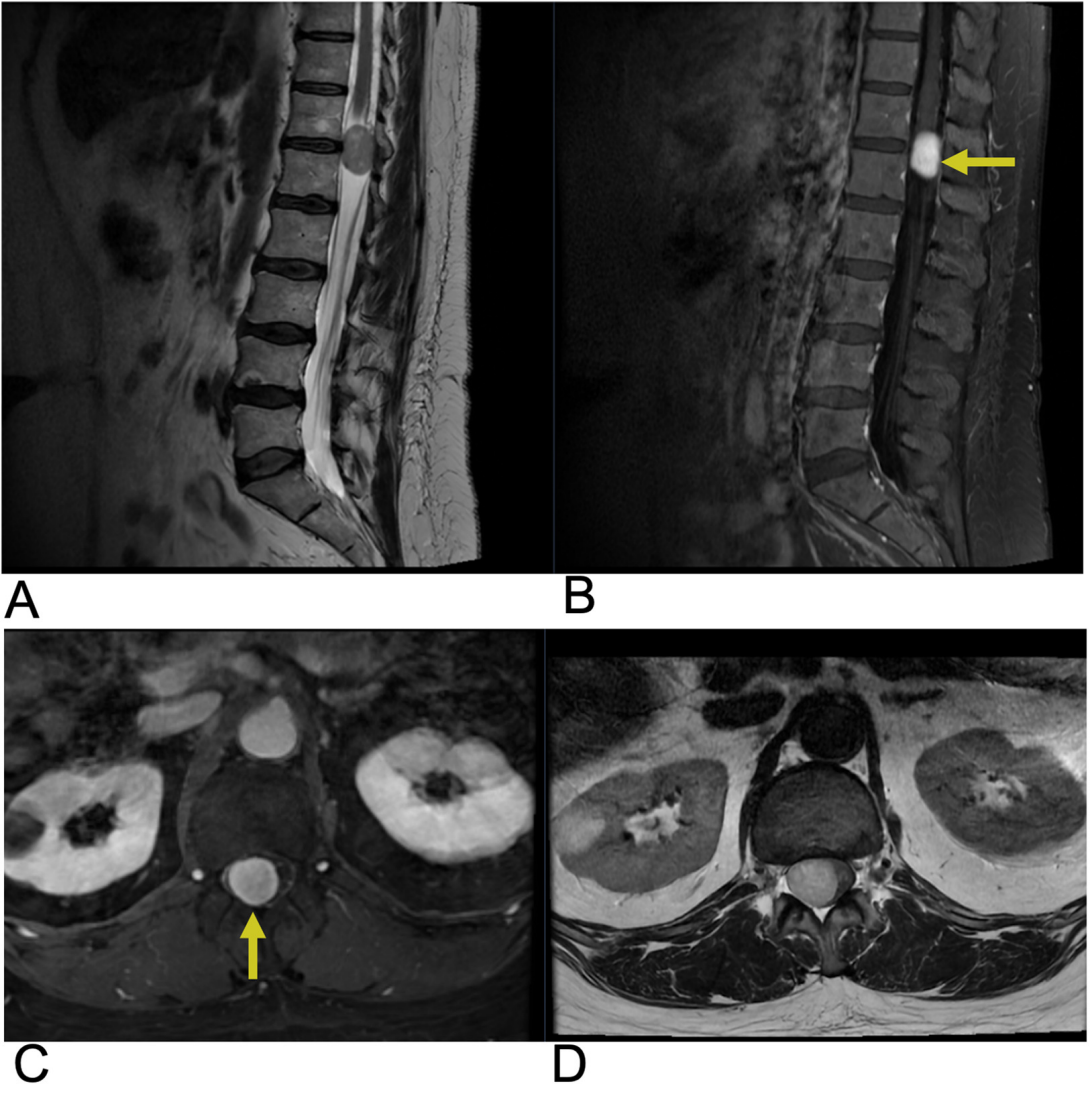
Schwannoma. Sagittal T2 (A), sagittal T1 + C (B), axial T1 + C, axial T2 (D) MRI of the lumbar spine demonstrating an intradural, extramedullary enhancing foci (yellow arrows) at the T12 level representing a biopsy-proven schwannoma causing mass effect affecting the spinal cord.
